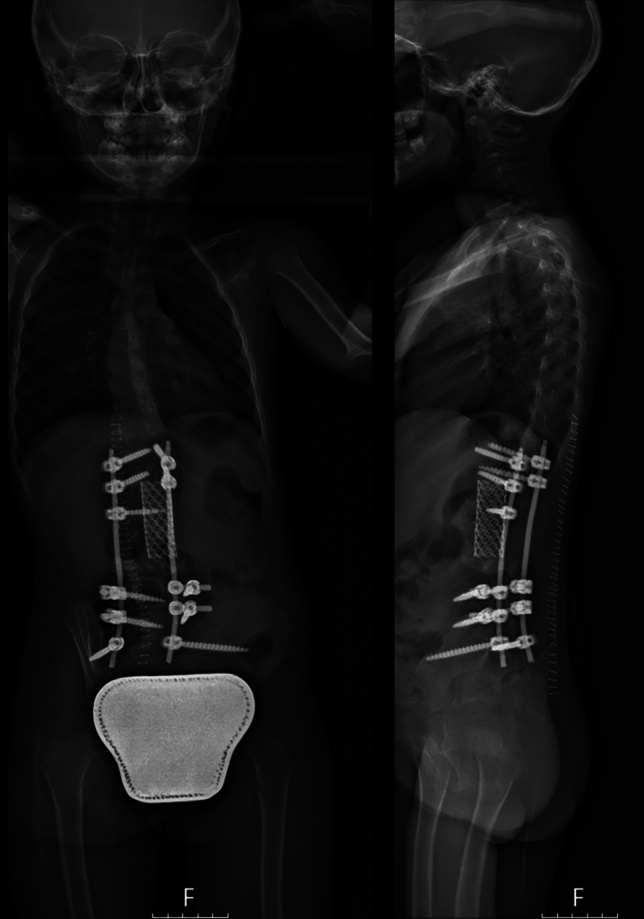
Post-operative X-ray showing posterior reconstruction with rods and screws; the anterior column was reconstructed with a titanium cage filled with autograft and homograft bone
